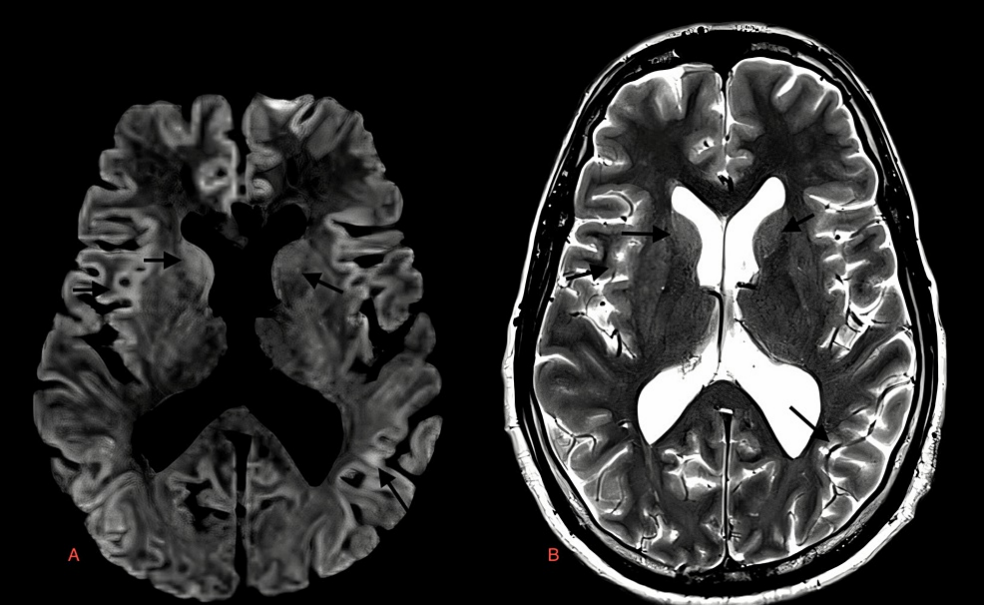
Case 3: DWI and T2W images with diffusion restriction and axial hyperintensities, respectively, in both cerebral hemispheres and both caudate nuclei (black arrows in A & B, respectively), MRI of a 36-year-old male patient with dengue encephalitis. DWI: diffusion-weighted image; T2W: T2-weighted image; MRI: magnetic resonance imaging
